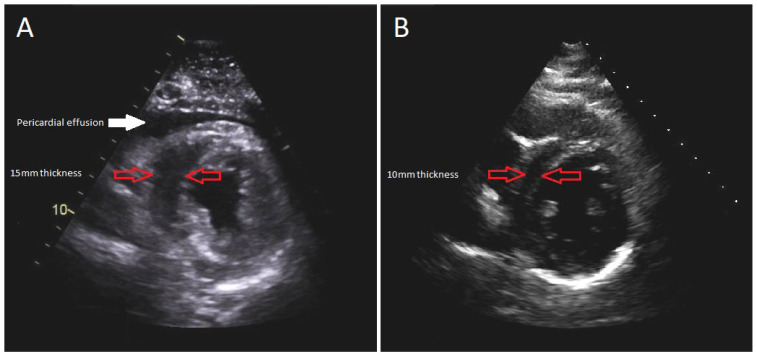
Parasternal short-axis view of TTE: (A) during the acute illness revealing 15 mm LV wall thickness; (B) 1-month follow-up demonstrating 10 mm LV wall thickness.
